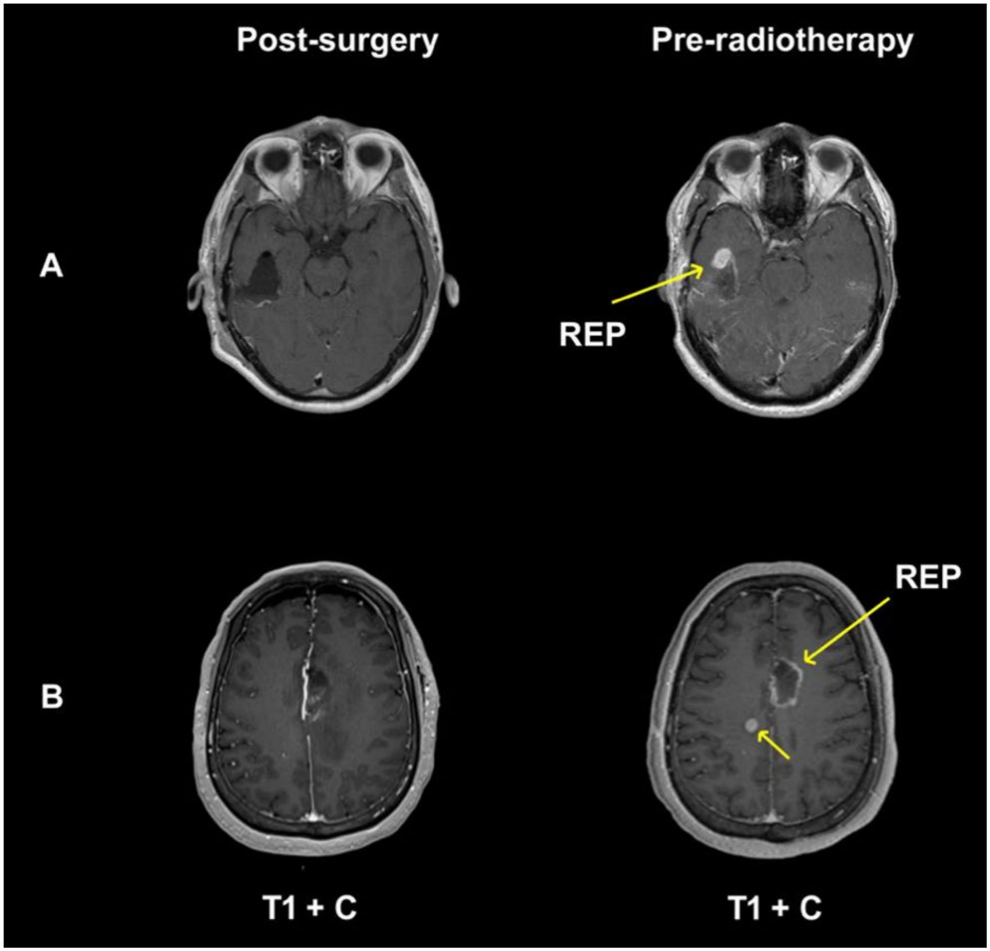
T1 gadolinium enhanced MRIs of two sample patients with newly diagnosed glioblastoma who demonstrate rapid early progression after gross total resection. (A) 37-year-old male who underwent right temporal craniotomy (post-surgery top left) demonstrates REP on pre-RT MRI (top right) with nodular lesion extending anteriorly and inferiorly along resection cavity. (B) 64-year-old woman who underwent left frontal craniotomy (bottom left) demonstrates REP on pre-RT MRI (bottom right) with recurrence of tumor along the posterior surgical cavity and new right posterior frontal centrum semiovale lesion measuring 1 cm
